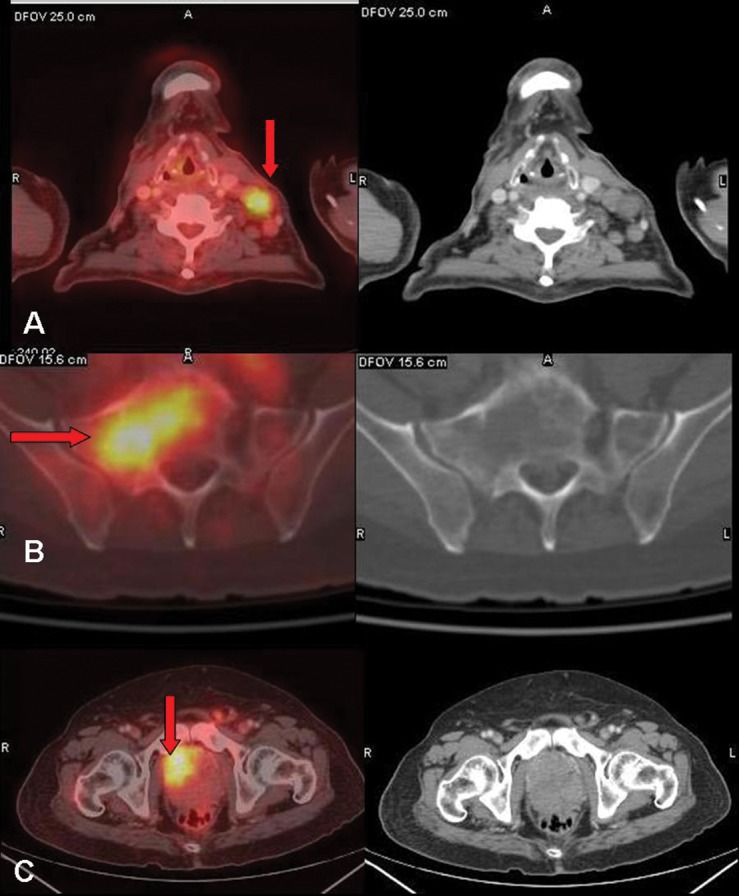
Transaxial Fused FDG PET/CT and CT Images. Figure 1A shows increased FDG uptake in the enlarged left cervical lymph nodes (Arrow), in a lytic lesion in the sacrum (Figure1B arrow), and in the heterogeneously enhancing right lobe of the enlarged prostate gland (Figure1C arrow) with a size of 7.5 × 7.5 cm and maximum standardized uptake value (SUVmax) of 5.5 gm/mL. These findings were indicative of a neoplastic process. TRUS-guided biopsy of the prostate lesion indicated a prostatic adenocarcinoma.
